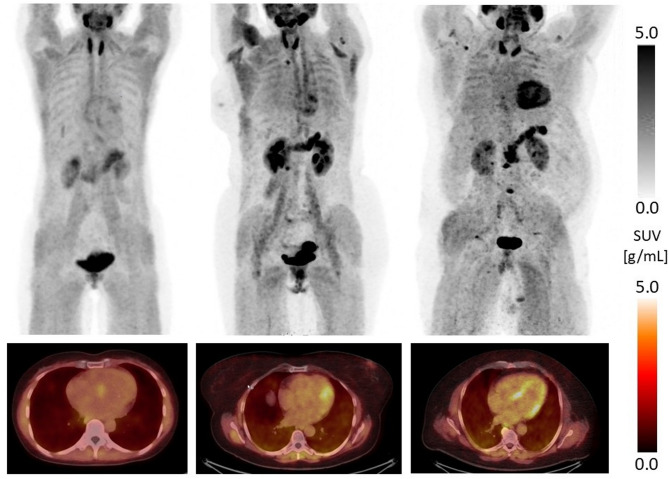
Highly variable patterns of FAP-uptake in PET maximal intension projection (upper row) and fused PET/CT images (lower row) in the left ventricular myocardium in different patients, ranging from no uptake at all (left), partial uptake in some regions (middle) to increased uptake over the whole left ventricular myocardium (right). FAP: fibroblast activation protein; SUV: standardized uptake value
